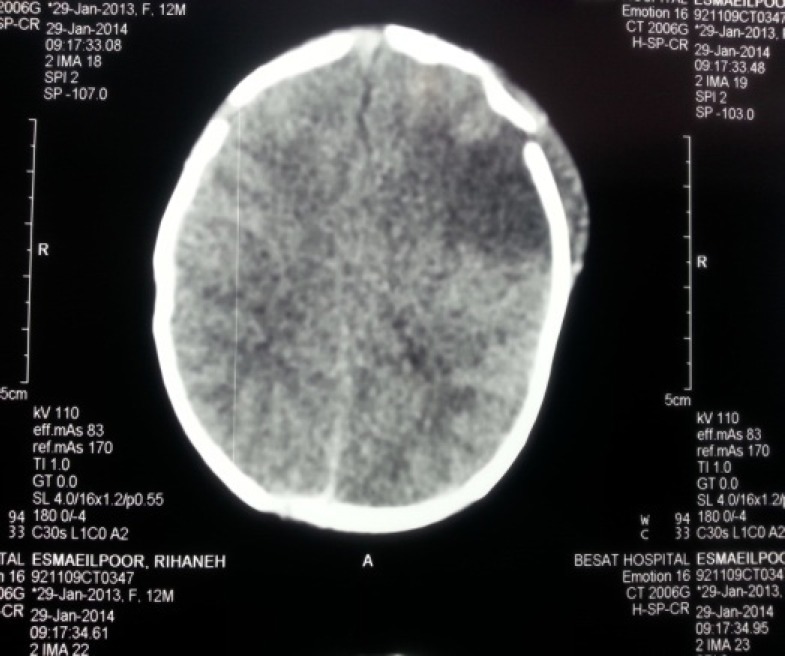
Unenhanced CT, performed on day 6, demonstrates a hypodense area in the anterior part of the left middle cerebral artery territory in the left frontoparietal region related to local ischemic changes. Adjacent cephalhematoma was also present.
